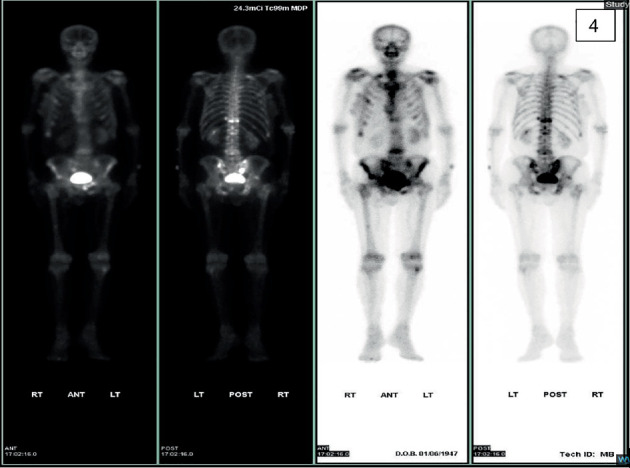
Whole-body bone scintigraphy demonstrating multiple foci of increased tracer uptake involving both axial and appendicular skeleton, consistent with widespread skeletal metastases.
